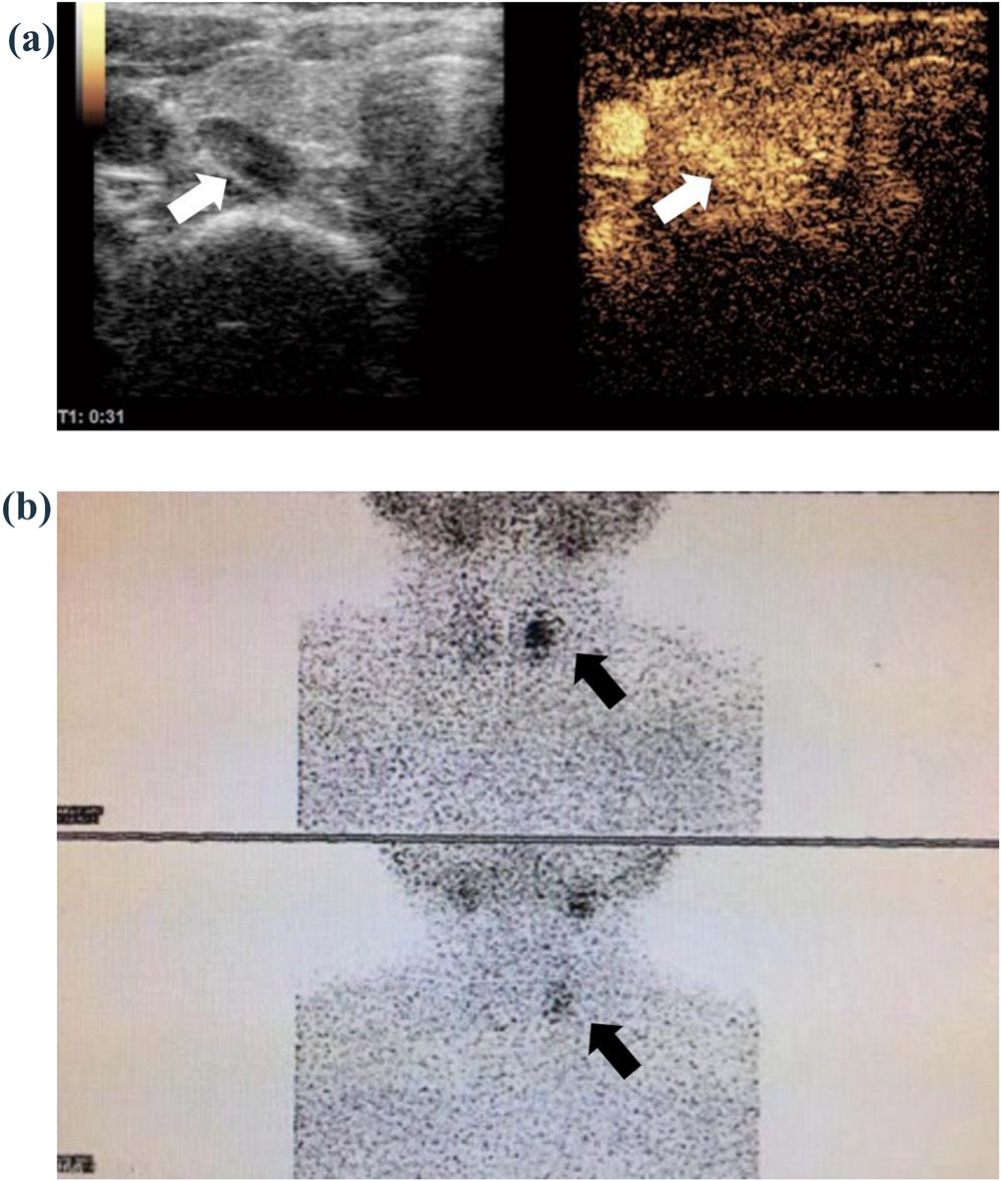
A 49‐year‐old female patient with HPHT. (a) Ultrasonography (US) shows a hypoechoic nodule behind the left thyroid lobe and contrast‐enhanced ultrasound (CEUS) shows high enhancement in the arterial phase (white arrow); (b) ^99m^Technetium‐sestamibi scintigraphy (^99m^Tc‐MIBI) scintigraphy shows a positive nodule in the left lobe of the thyroid (black arrow).
